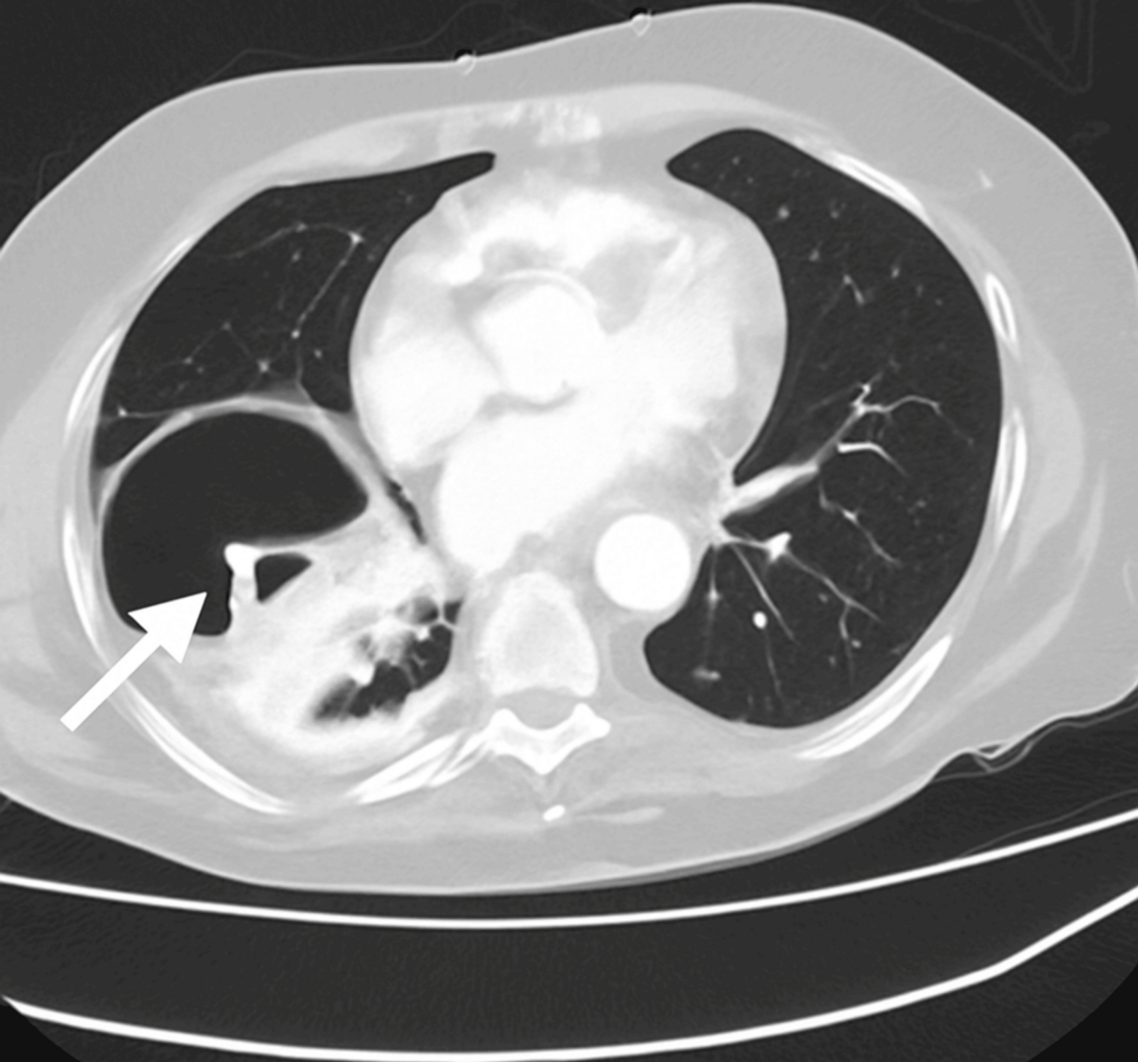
Axial CT scan of the chest revealing a large right-sided intrathoracic air-filled structure with internal soft tissue density, consistent with herniated bowel loops. The bowel wall and mucosal folds are clearly visible within the thoracic cavity, delineating the presence of abdominal contents that have passed through a diaphragmatic defect. The arrow highlights this sharply marginated structure—representative of a segment of small intestine—displacing adjacent lung parenchyma and confirming the diagnosis of a right-sided diaphragmatic hernia. This CT scan was obtained after chest drain placement and played a pivotal role in correcting the initial misdiagnosis of hydropneumothorax.
